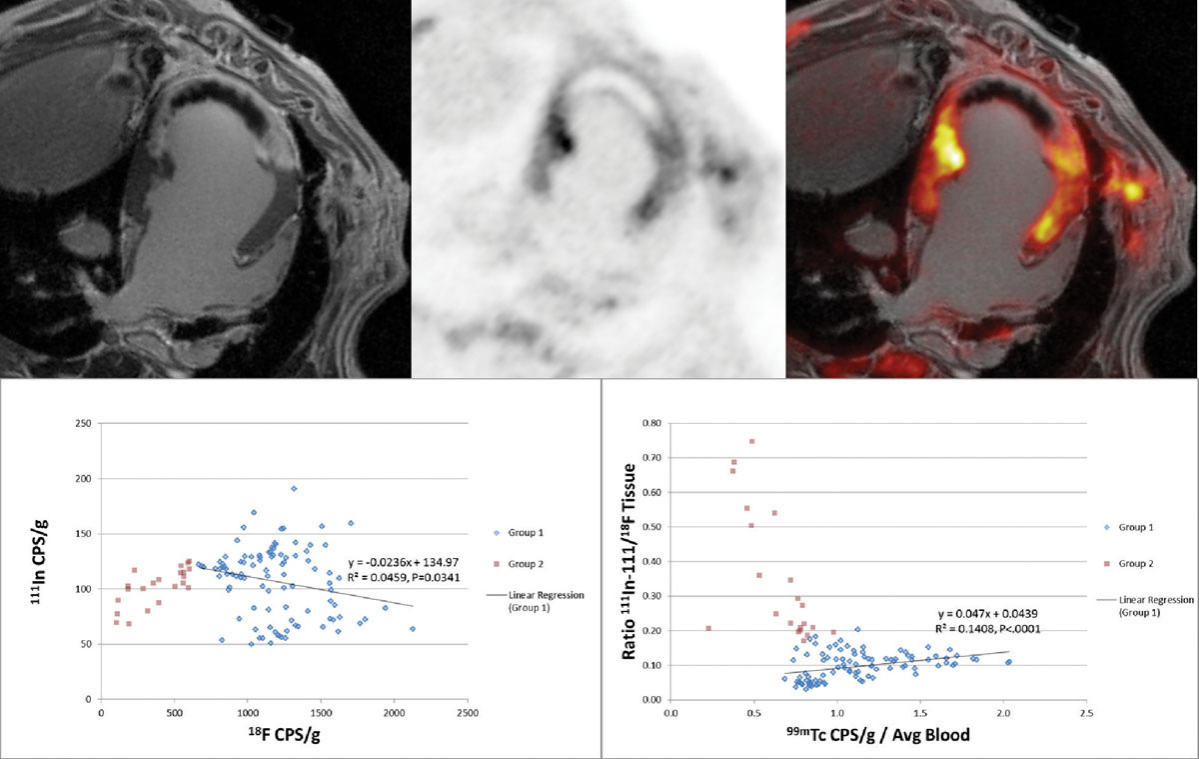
Post-mortem PET/MRI images from subject with permanent LAD occlusion imaged 7 days post MI. The images demonsrate both enhanced Gd-DTPA and FDG uptake surrounding a large central zone of microvascular obstruction (MO), suggesting minimal inflammatory cell activity in that region. However, analysis of the ex vivo tissue demonstrates a dissociation between Indium and FDG concentrations in tissue obtained from the MO zone. In some samples, Indium levels were up to 7 times higher, suggesting penetration of the labeled white blood cells injected 24 hours earlier, but minimal FDG following a single bolus injection. Therefore, FDG imaging may signficantly underestimate the degree and extent of inflammatory cell activity in the setting of MO.
